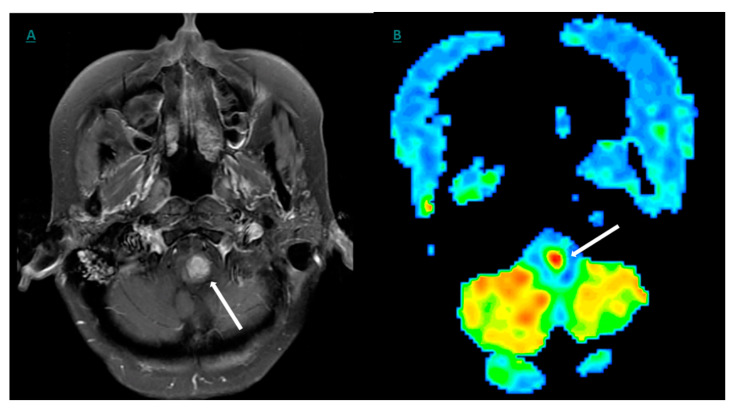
(A) DCE MRI axial T1-weighted image showing an enhancing cervicomedullary junction lesion (arrow). (B) The corresponding Arterial Spin Labeling (ASL) showing a relatively increased perfusion in the cervicomedullary junction (arrow).
